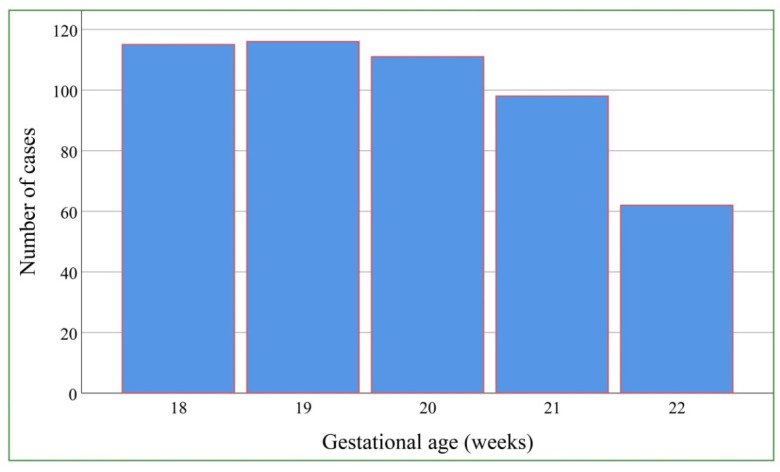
Distribution of gestational age at the time of ultrasound diagnosis.
